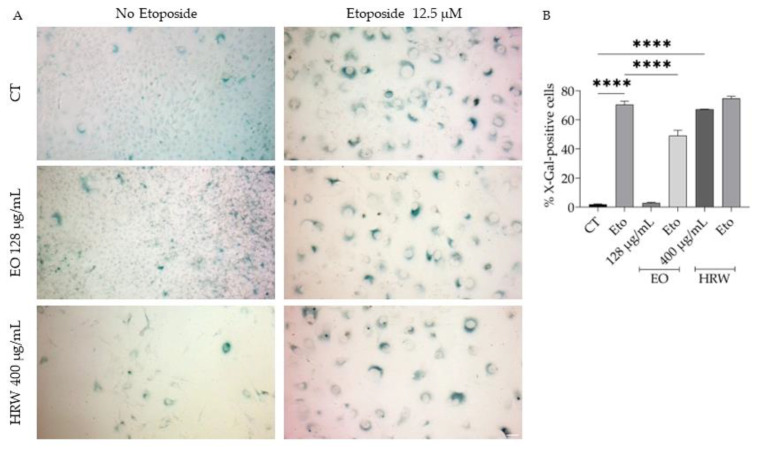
Effect of *T. capitata* EO and HRW on etoposide-induced cellular senescence. Representative bright-field images (A) and percentage of X-gal-positive cells after the mentioned treatments (B).  *p* < 0.0001 when compared to control (CT) or etoposide (Eto) after one-way ANOVA followed by Dunnett’s multiple comparison test.
